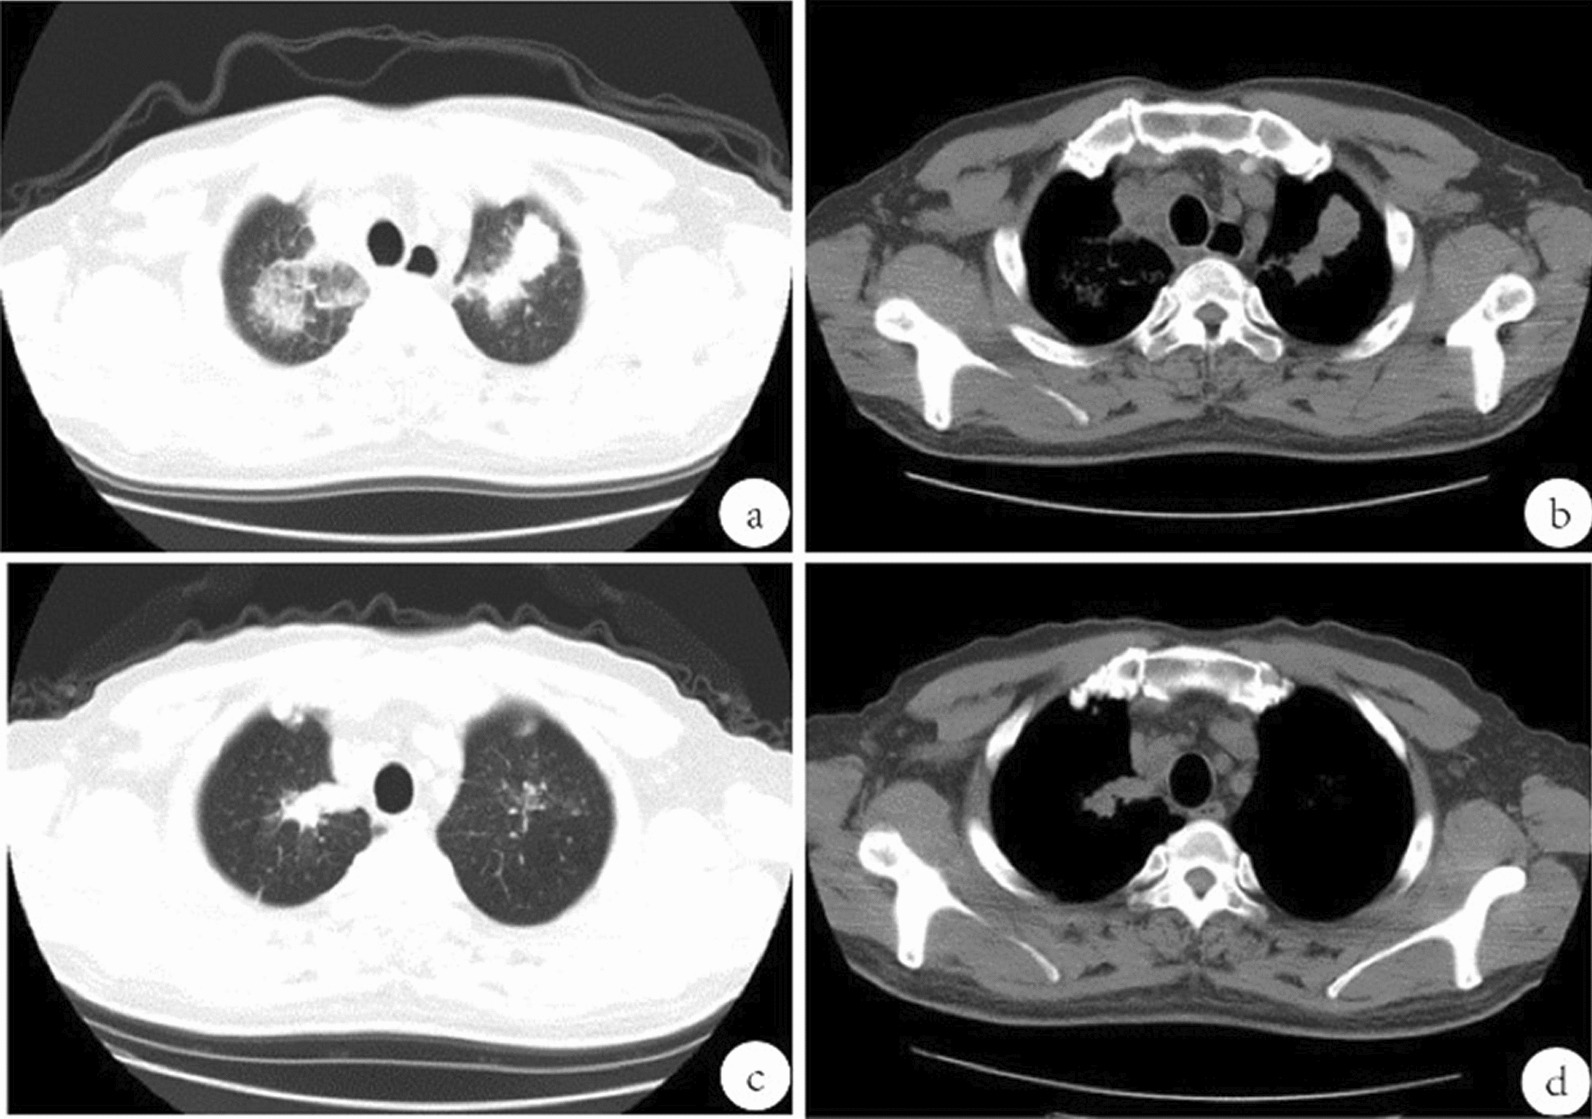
Chest computed tomography performed at different times. a, b Computed tomography image of the patient after admission to the hospital on hospitalization day 1 due to fever. c, d Computed tomography image 12 days after discharge
